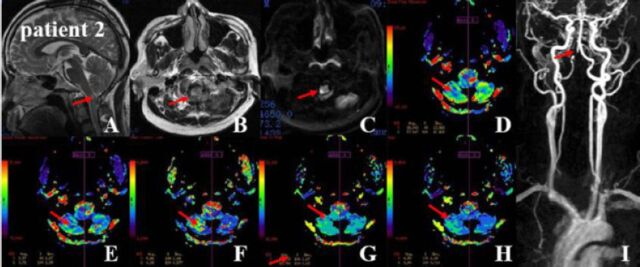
MRI-T2WI, Flair and DWI demonstrated a-c) high signal intensities in the right lateral medulla. d-h) Dynamic susceptibility contrast-enhanced-perfusion weighted imaging demonstrated a right ROI low CBF, low CBV, delayed TTP, delayed MTT, and delayed time maximum on the VAH side in Case 2. The representative rigid quantitative thresholds in the ROIs of 5 and 6 are shown in Table 2. i) constrast-enhanced megnetic resonance artery indicated right VAH and severe stricture and dilated region in the V4 segment of the right vertebral artery and moderate kinking of the left vertebral artery. VAH - vertebral artery hypoplasia, CBF - cerebral blood flow, CBV - cerebral blood volume, TTP - time to peak, MTT - mean transit time
